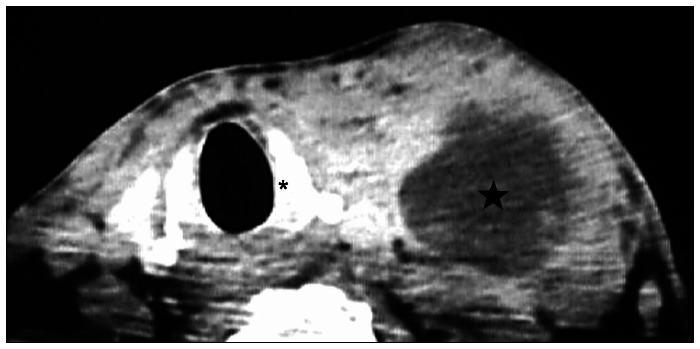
Axial computed tomography scan at the cricoid cartilage (*) level. Normal appearance of subglottis. True-negative example. The scan shows left lymph node enlargement (star).
